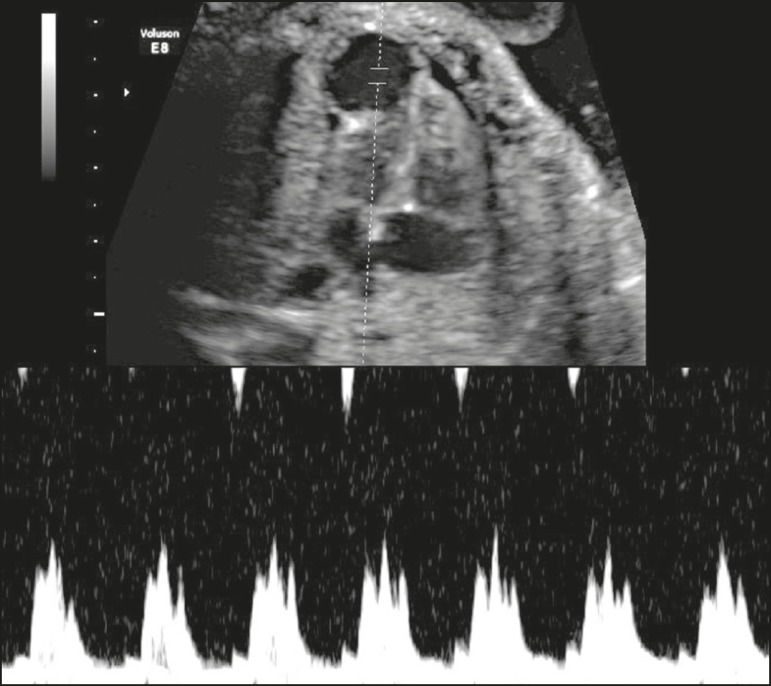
Spectral Doppler ultrasound showing triphasic flow and high pulsatility
